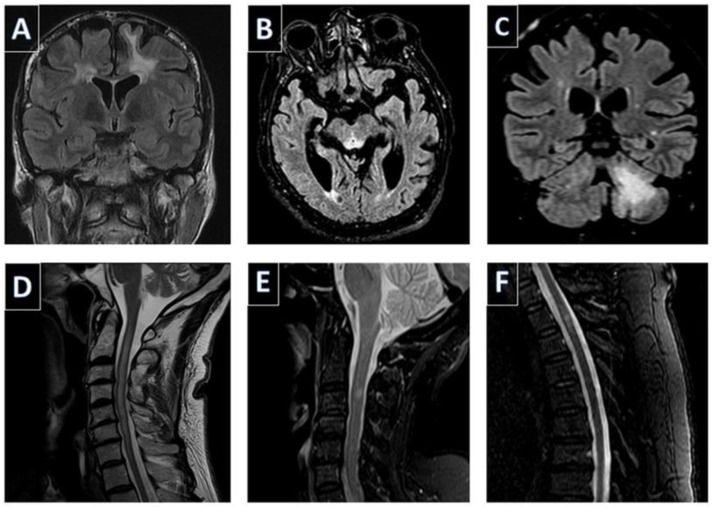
Representative images from brain (A–C) and spinal cord (D–F) MRI scans in aged people with secondary demyelination. (A) Coronal FLAIR image demonstrating diffuse T2 hyperintensities in a 68-year-old female with postradiation demyelination. (B) Axial FLAIR image demonstrating T2 hyperintensity in the periaqueductal area, in a 74-year-old male with Wernicke encephalopathy. (C) Coronal FLAIR image demonstrating a diffuse T2 lesion in the cerebellum, in a 71-year-old female with progressive multifocal leukoencephalopathy. (D) Sagittal T2-weighted image of the cervical spine demonstrates a postinfectious longitudinal extensive myelitis in a 65-year-old male. (E) Sagittal T2-weighted short-tau inversion recovery image of the cervical spine of a 66-year-old female with myelitis attributed to anti-TNF agents. (F) Sagittal T2-weighted image revealing a T2 lesion in the upper thoracic spinal cord of a 67-year-old female with myelitis attributed to cyclosporin.
